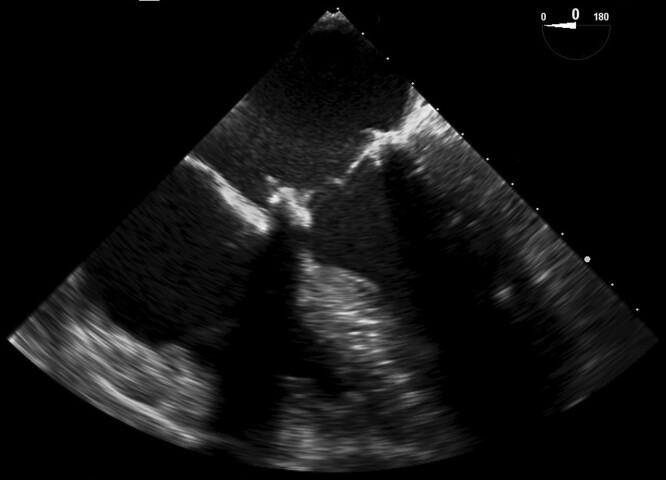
Peri-operative transoesophageal echocardiography mid-oesophageal four-chamber (ME 4C) view showing replaced mitral valve with bioprosthesis.
